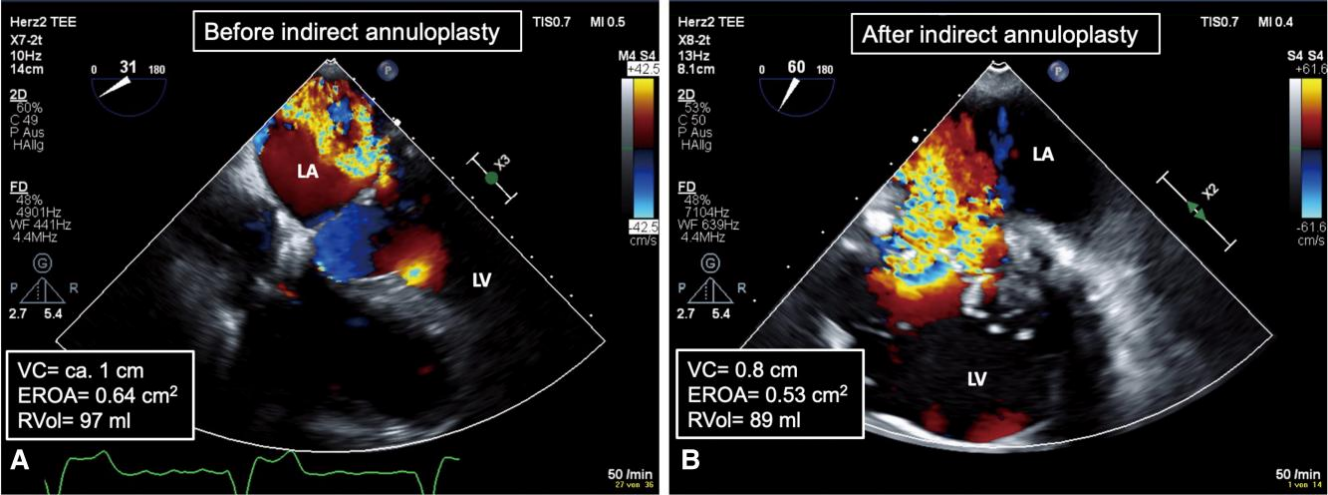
Pre-operative 2D TEE with colour Doppler images before and after Carillon device implantation. (*A*) Presence of severe MR prior to the indirect annuloplasty procedure. (*B*) Unfavourable outcome of the procedure, characterized by severe and persistent MR. 2D TEE, two-dimensional transoesophageal echocardiography; VC, vena contracta; MR, mitral valve regurgitation; EROA, effective regurgitant orifice area; R-Vol, mitral valve regurgitant volume.
